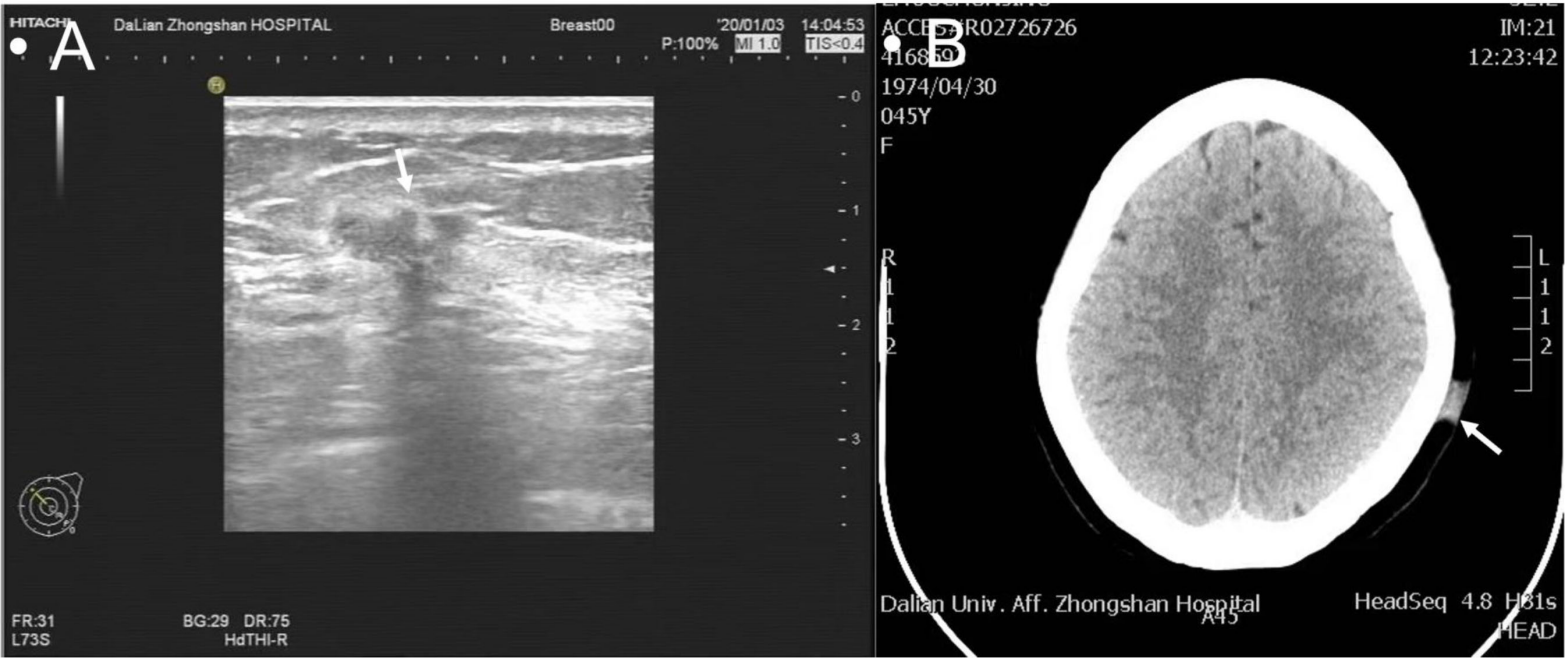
Multiple nodular masses with calcifications on ultrasound classified as BI-RADS 6 (A); a 1.3-cm hyperdense patchy lesion on CT scan (B).
